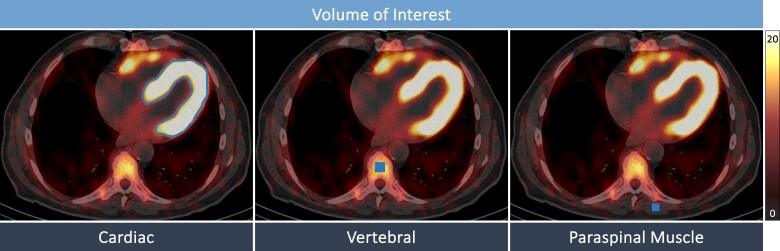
DPD quantification. DPD SPECT/CT images of a representative ATTR-CA patient demonstrating the VOI for each tissue (VOI cardiac: automatically generated using a threshold-based method, VOI vertebral: 2.92 mL, VOI paraspinal muscle: 1.19 mL) placed to quantify the SUV. ATTR-CA, transthyretin cardiac amyloidosis; DPD, [^99m^Tc]-3,3-diphosphono-1,2-propanodicarboxylic acid; SPECT/CT, single-photon emission computed tomography/computed tomography; SUV, standardized uptake value; VOI, volume of interest.
